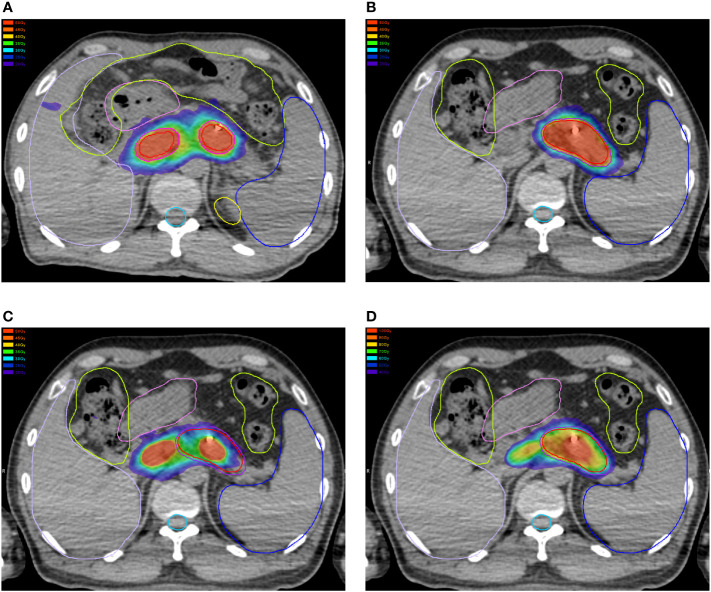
Dose distributions in the (A) first and (B) second plan. (C) Dose distributions in the first plan projected to the second CT scans. (D) Summations of dose distributions of the first and second plan by deformable image registration.
